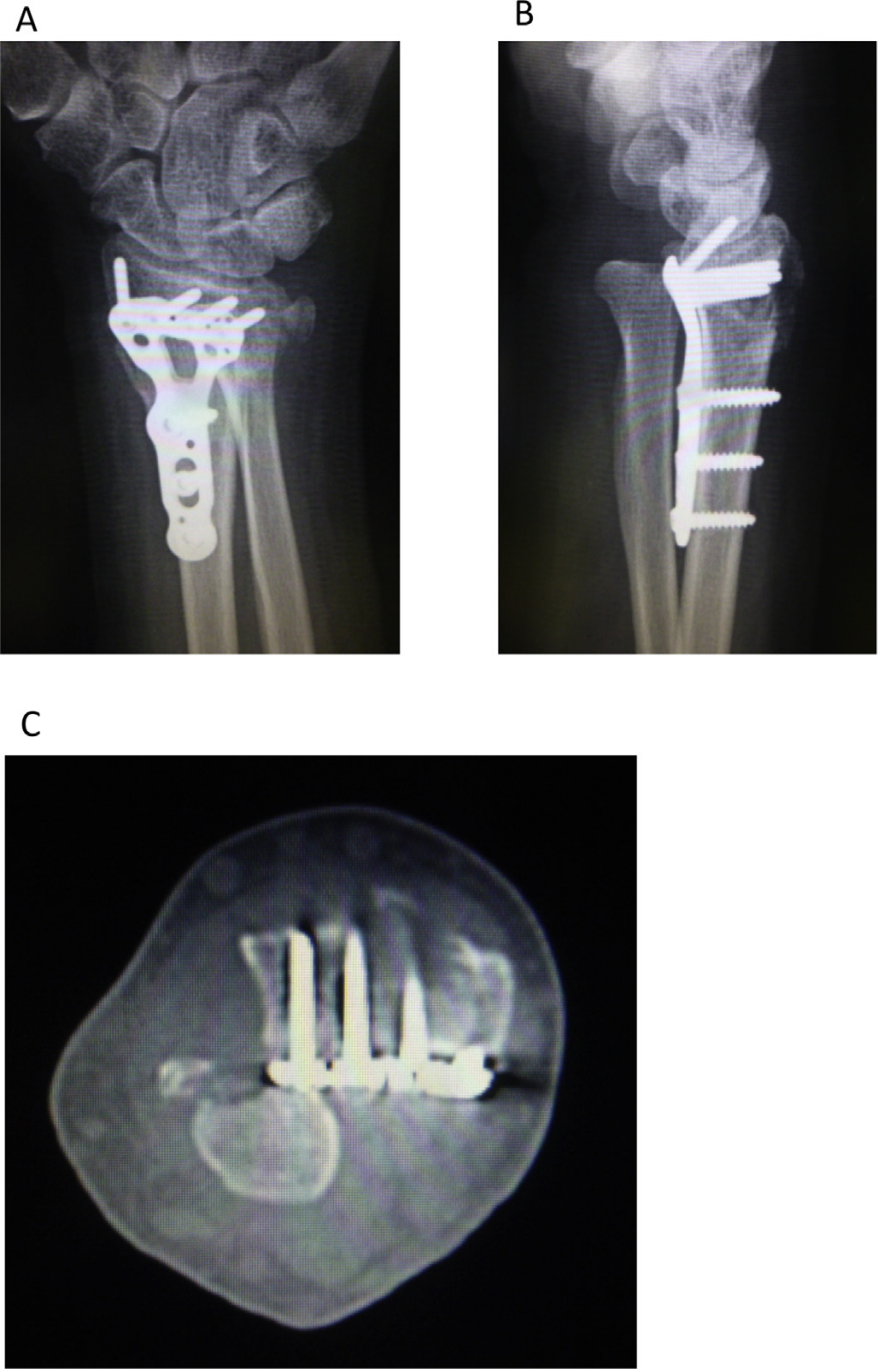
Plain radiography and CT 2 weeks after the first surgery. On plain radiography, incongruency of DRUJ and deviation of the ulnar head were noted (A: frontal view, B: lateral view). With an axial view on CT, volar dislocation of the ulnar head was noted (C).
